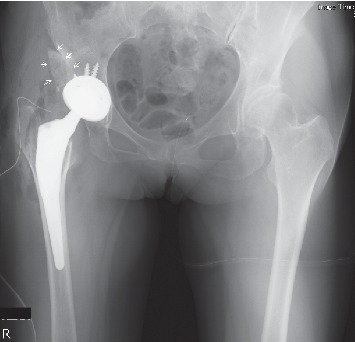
Anteroposterior radiograph immediately after total hip arthroplasty. A bulk bone was grafted by bioabsorbable screws.
